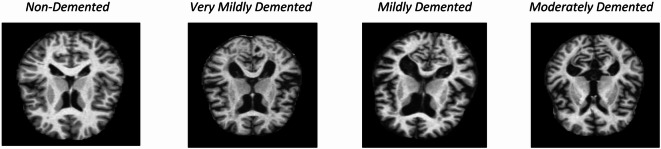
Sample MRI images from the four cognitive classes of the ADNI dataset.
